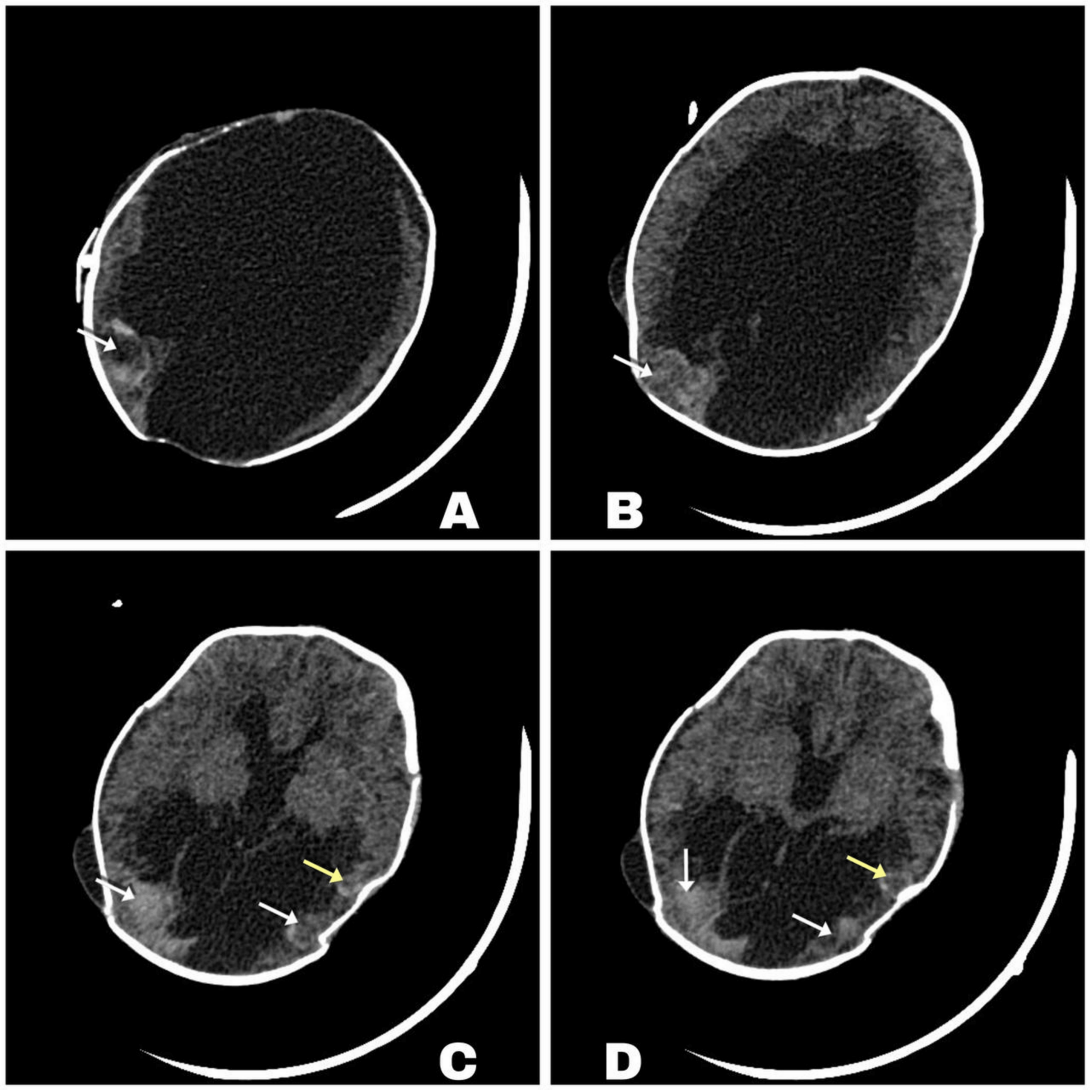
Follow-up CT scan of the brain A-D: White arrows indicate right occipito-parietal intraparenchymal hematomas and yellow arrows indicate left temporo-parietal intraparenchymal hematomas. CT: computed tomography
